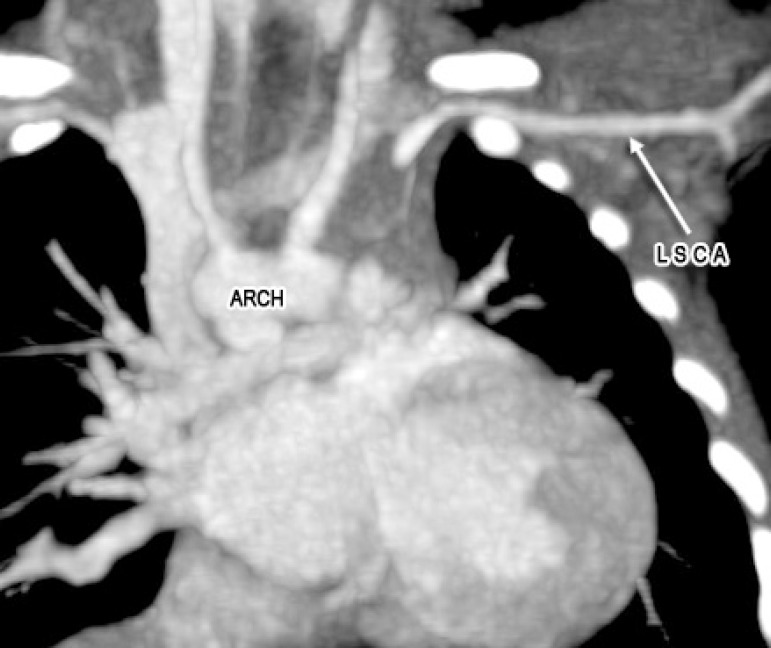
CT scan images showing isolated left subclavian artery arising from left pulmonary artery with thrombosed ductal tissue intervening them and filling by collaterals.
